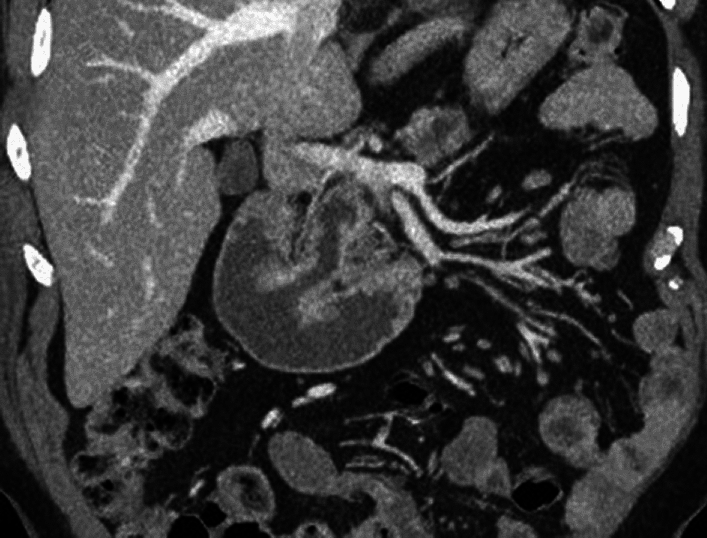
Main duct intraductal papillary mucinous neoplasm (MD-IPMN) with a ‘fish-mouth’ appearance of the ampulla of Vater seen on Magnetic resonance imaging (MRI) of the abdomen
